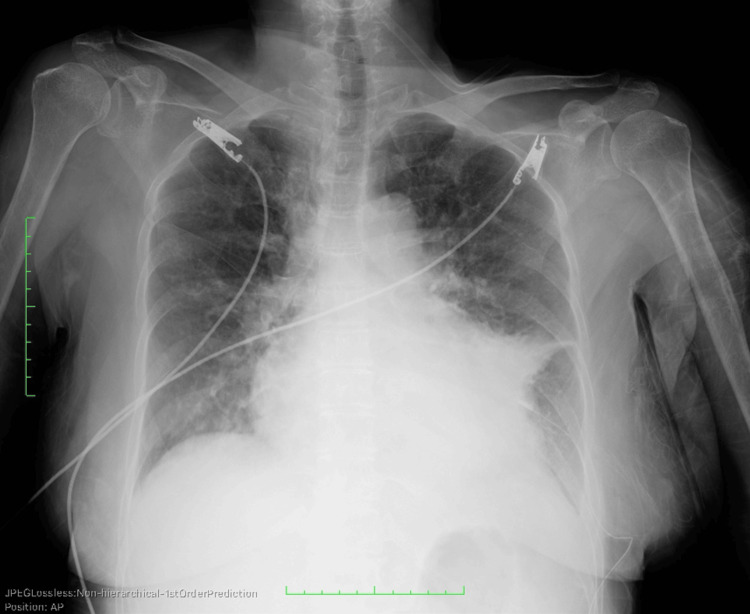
Chest radiography of the patient The image shows massive cardiomegaly and left pleural effusion.
